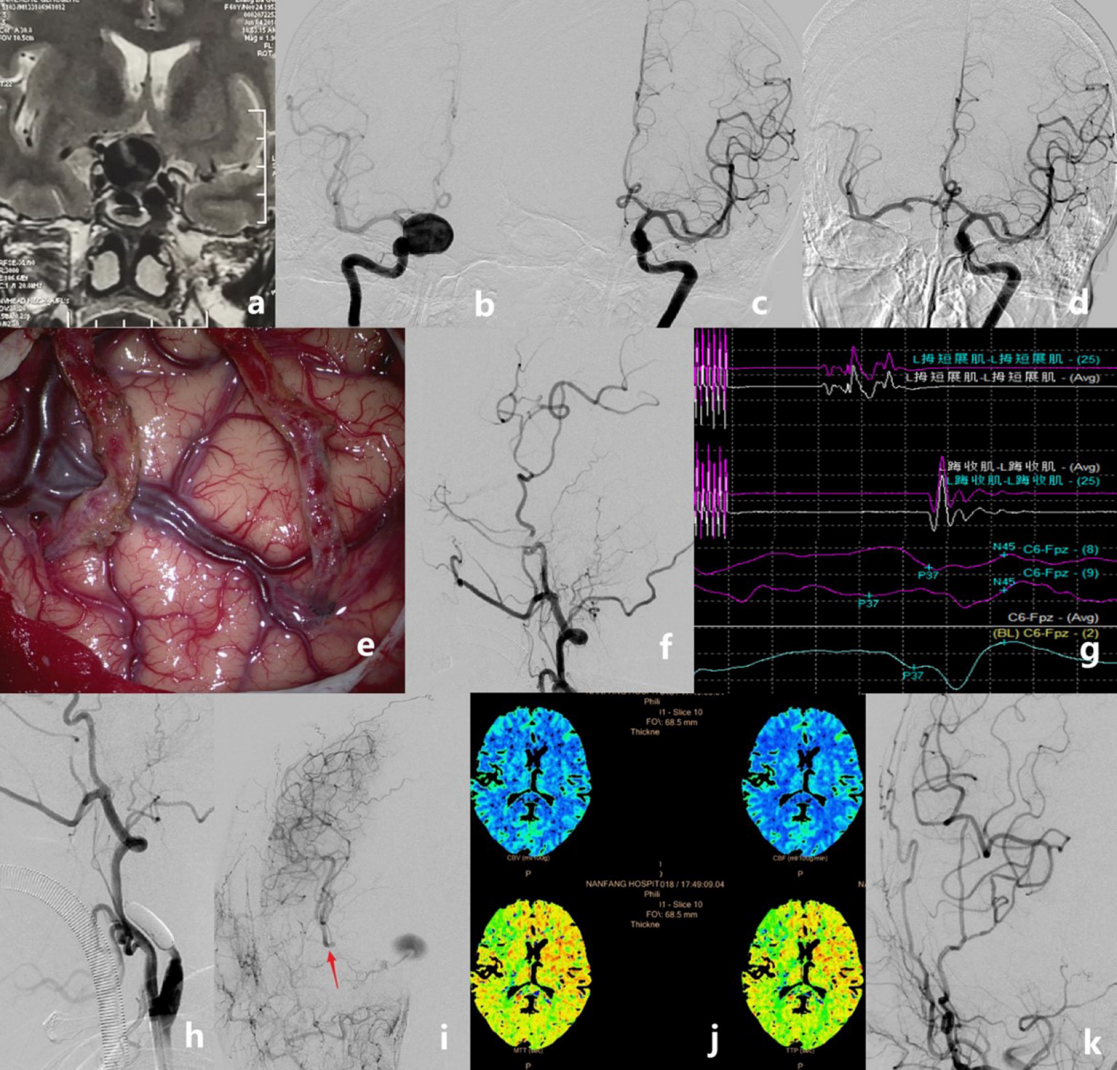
Case example of a patient who had a giant internal carotid artery (ICA) aneurysm. MRI showed a mass with fluid void effect in the sellar region (a). Pre-operative angiograms showing that the aneurysm was located at the right C4 [(b), anteroposterior view]. The anterior communicating artery did not show on regular left ICA angiogram [(c), anteroposterior view]. Pre-operative balloon occlusion test showing the anterior communicating artery and delayed filling of the right ICA territory [(d), anteroposterior view]. Double superficial temporal artery–middle cerebral artery (STA–MCA) bypass was performed (e). Intraoperative angiography showing the good patency of the anastomoses (f). The intra-operative motor evoked potential and somatosensory evoked potential remained stable after temporary occlusion of the ICA for 30 min (g). The ICA was occluded permanently with detachable balloons (h). Controlled angiography showing the good patency of the double STA bypass and the MCA territory that was compensated very well, but the aneurysm was still stasis and fed by the anastomosic artery from the external artery (i). Post-operative perfusion CT showed no ischemic area on the right hemisphere (j). Digital subtraction angiography follow-up revealed the total obliteration of the aneurysms and the good patency of grafts (k).
